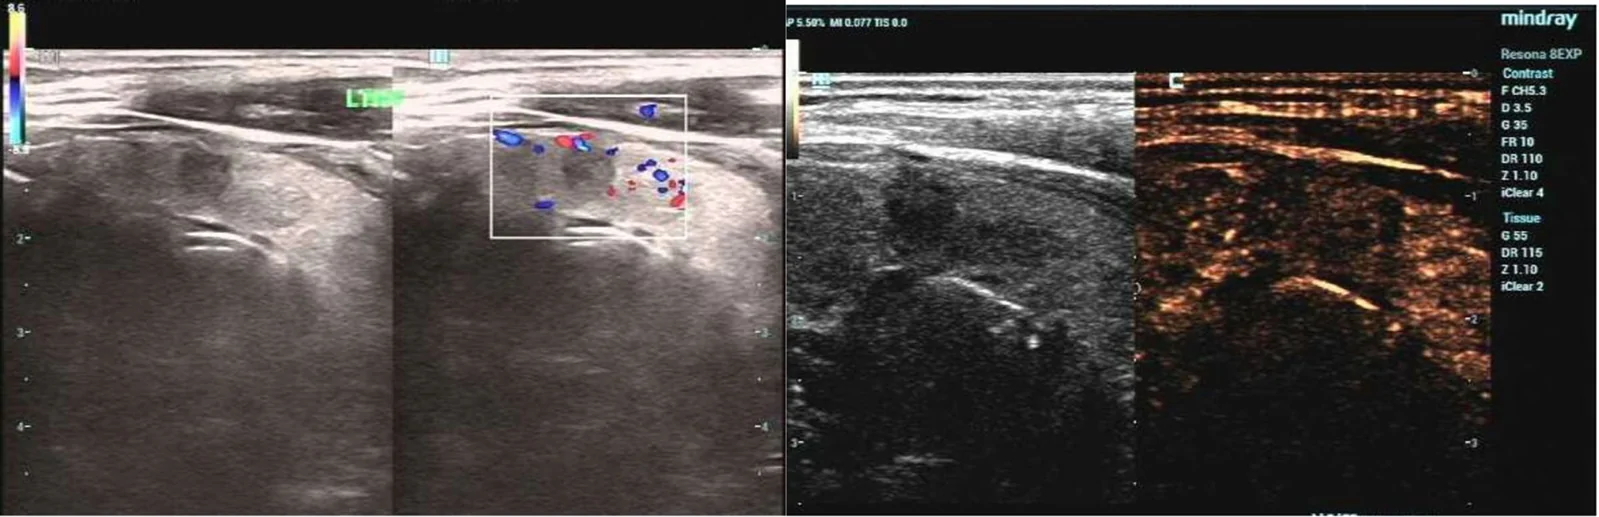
Contrast-enhanced thyroid ultrasound showed a hypoechoic mass in the left lobe of the thyroid and a mixed echo mass in the right lobe of the thyroid.
